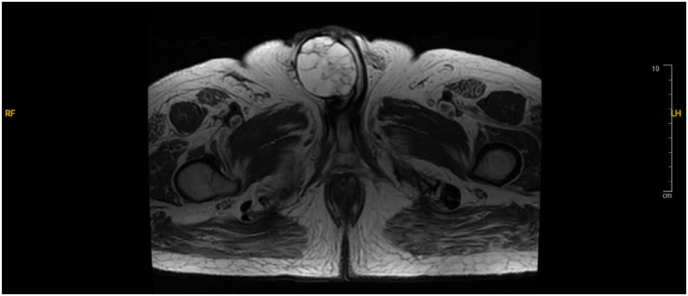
Axial T2 MRI pelvis revealed a septated mass at the base of the penis.
